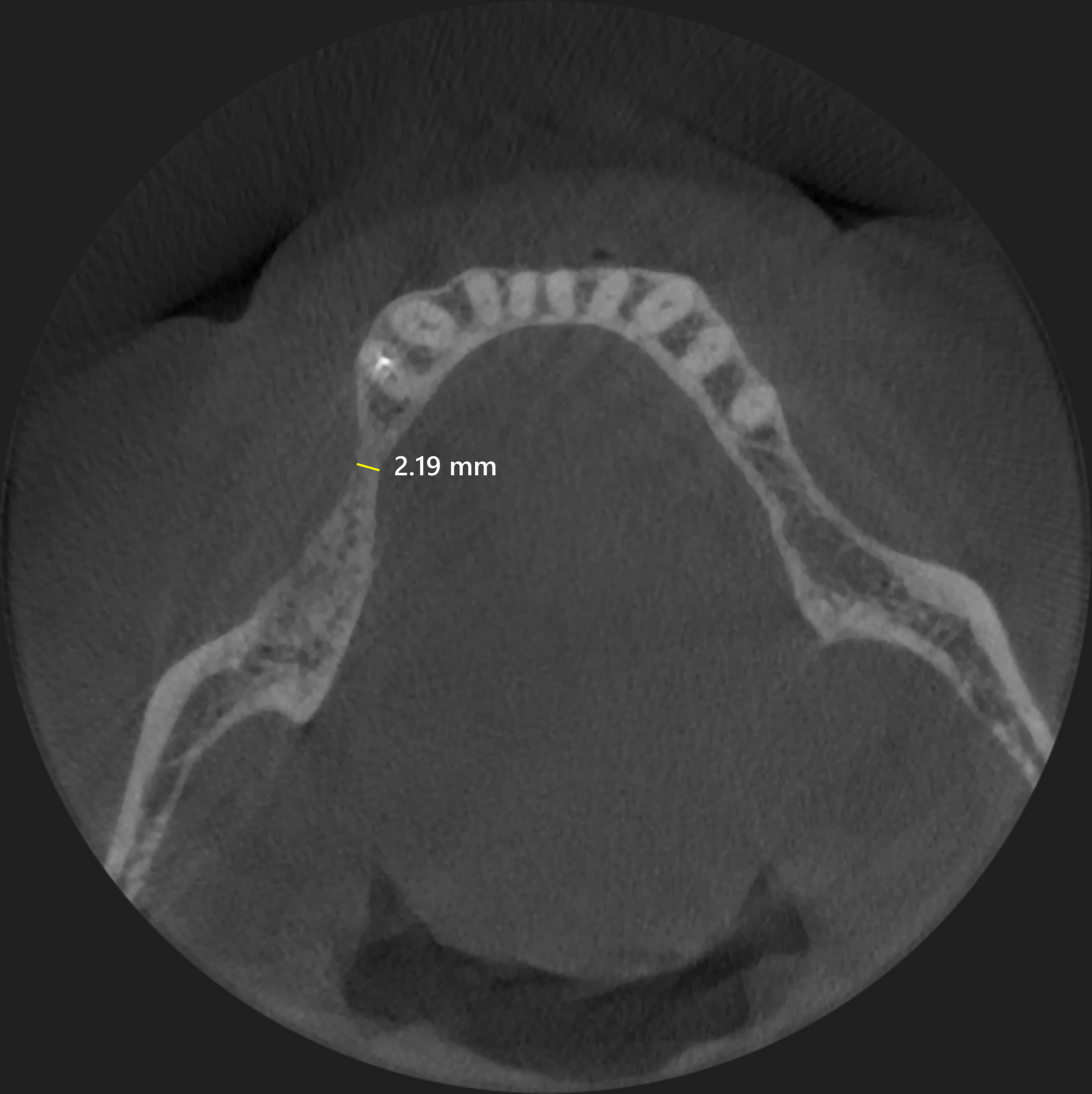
CBCT examination showing the minimal remaining thin crestal ridge (≈2.2 mm) in the fourth anatomical quadrant.
